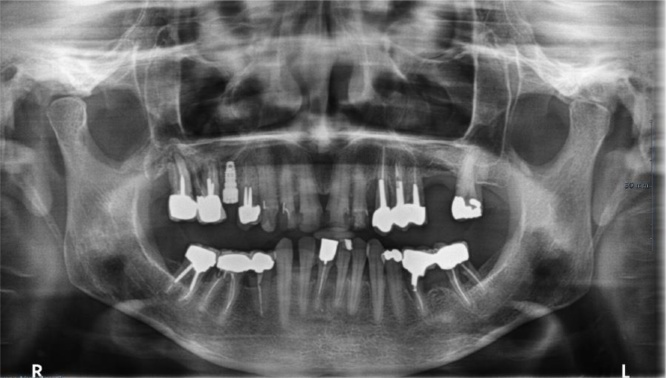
Initial orthopantomogram (case 1) showing maxillary (teeth number 14-16-17-23) or mandibular (teeth number 35-37-45-46) chronic infectious sites.
